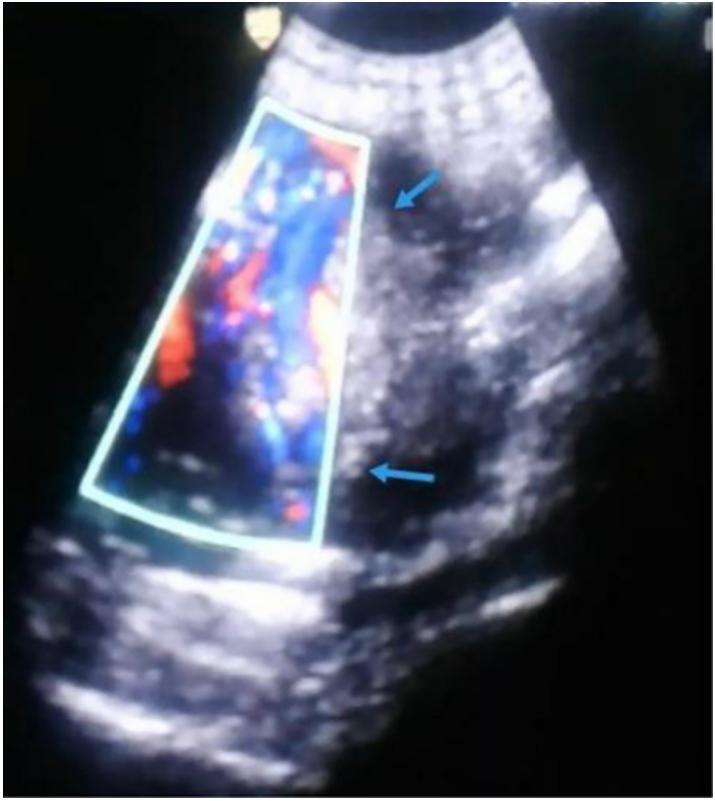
Doppler ultrasound shows placenta (blue arrows) with abnormal vascularization (increased and turbulent flow). (For interpretation of the references to colour in this figure legend, the reader is referred to the web version of this article.)
